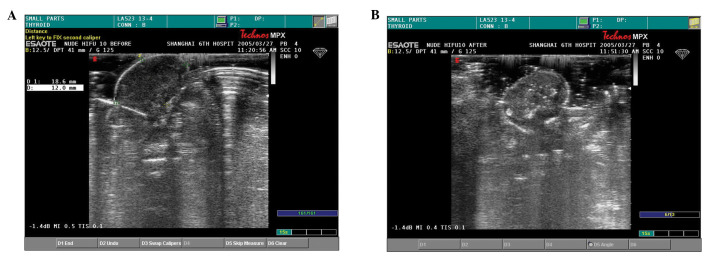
Ultrasound images of a tumor prior to and following HIFU treatment with an approximate volume of 0.1 cm^3^. (A) A tumor prior to HIFU treatment. Subcutaneous pancreatic cancer tumour appears as a hypoechoic area. (B) Treated tumour immediately after HIFU application. HIFU lesions appear as hyperechoic spots.
